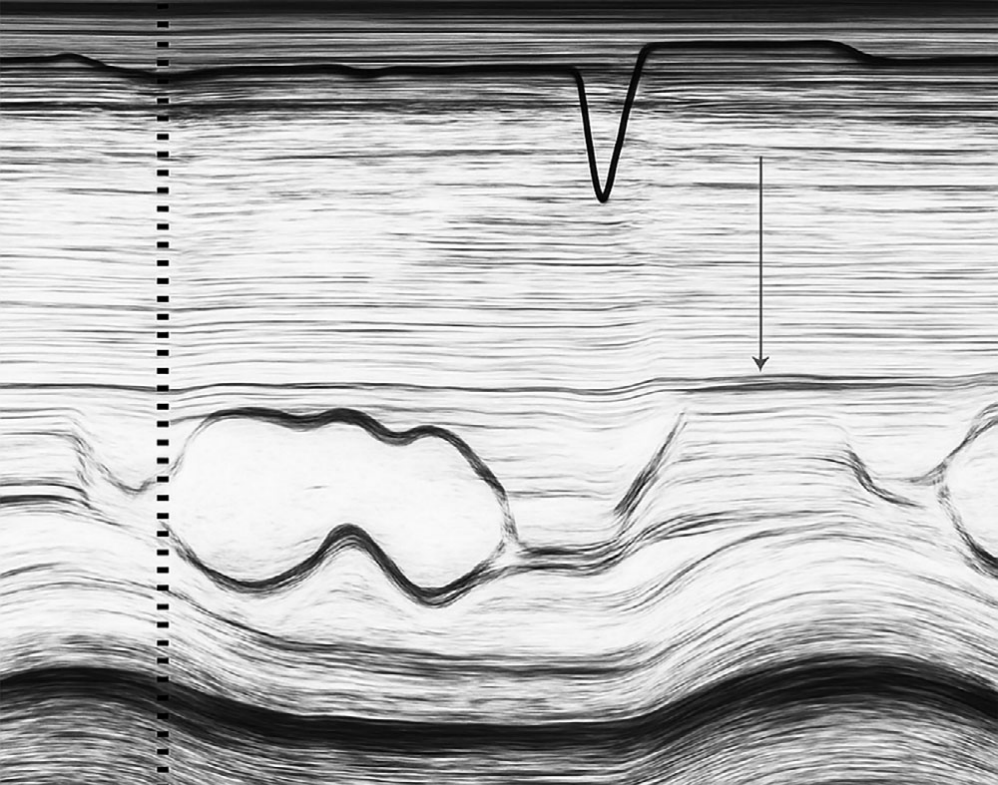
M‐mode echocardiogram demonstrating systolic anterior motion (SAM) of the mitral valve. The image captures the characteristic anterior displacement of the mitral valve leaflet during systole, indicative of hypertrophic obstructive cardiomyopathy (HOCM). The arrow marks the point of maximal anterior motion toward the interventricular septum, contributing to dynamic left ventricular outflow tract (LVOT) obstruction.
